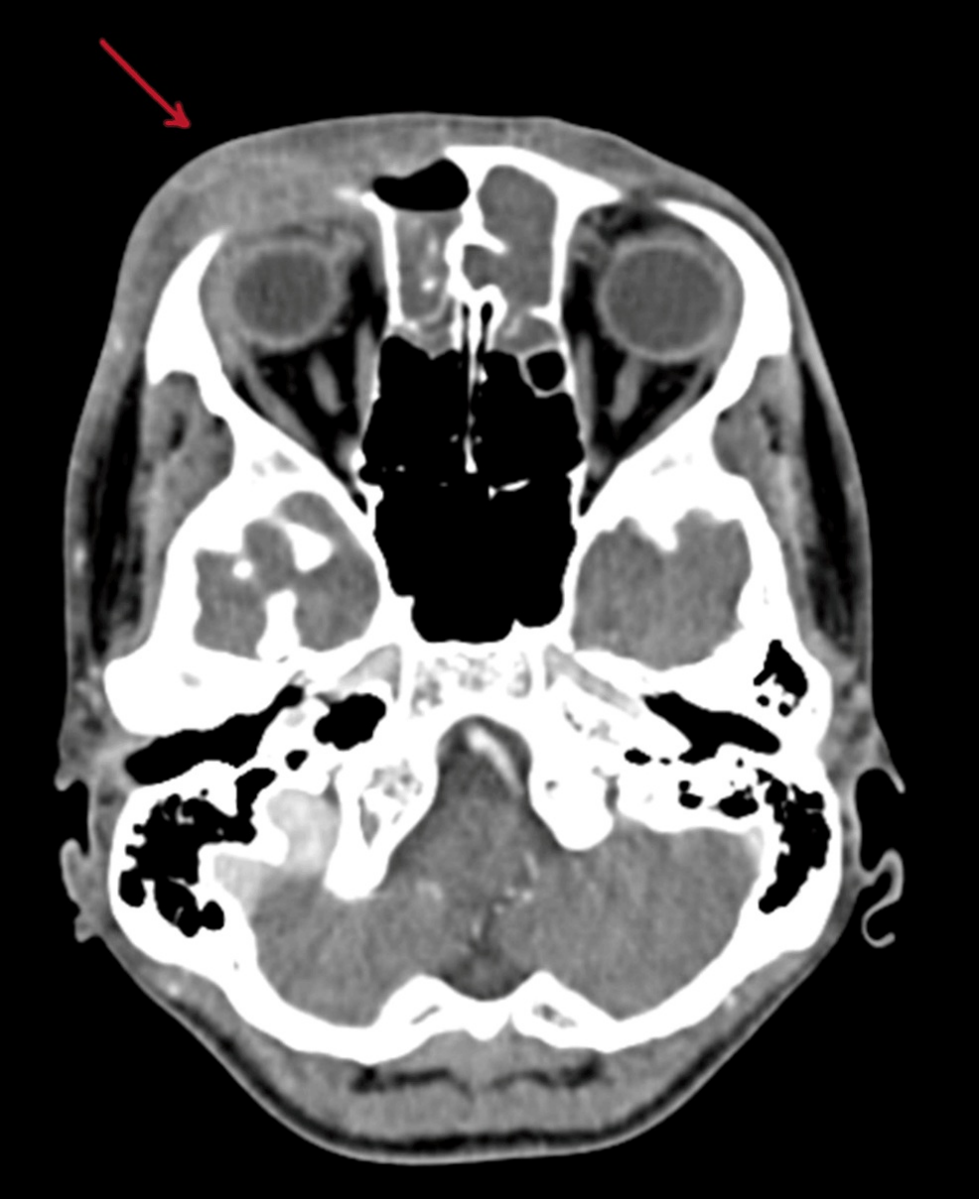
Contrast-enhanced CT showing periorbital cellulitis (indicated with red arrow).
